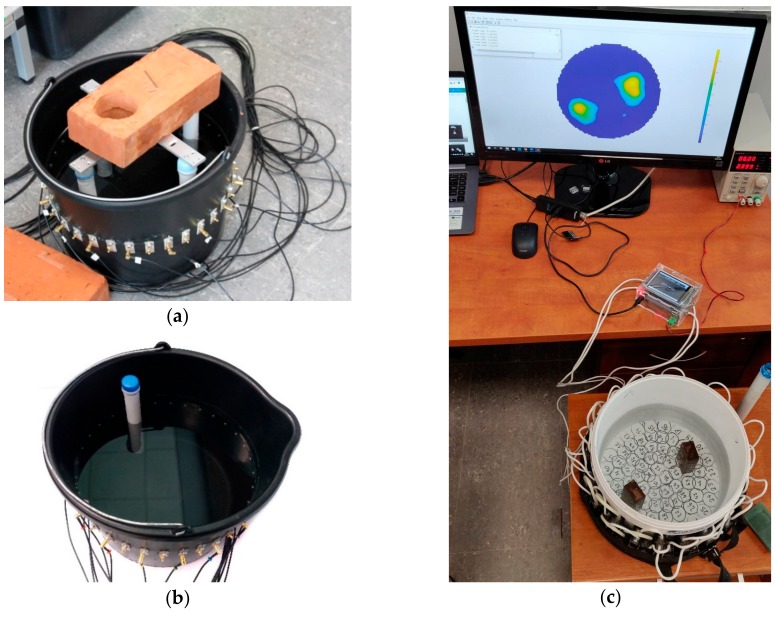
The physical model of the reactor with: (a,b) electrical impedance tomography (EIT) electrodes, (c) ultrasound transmission tomography (UST) transducers.
